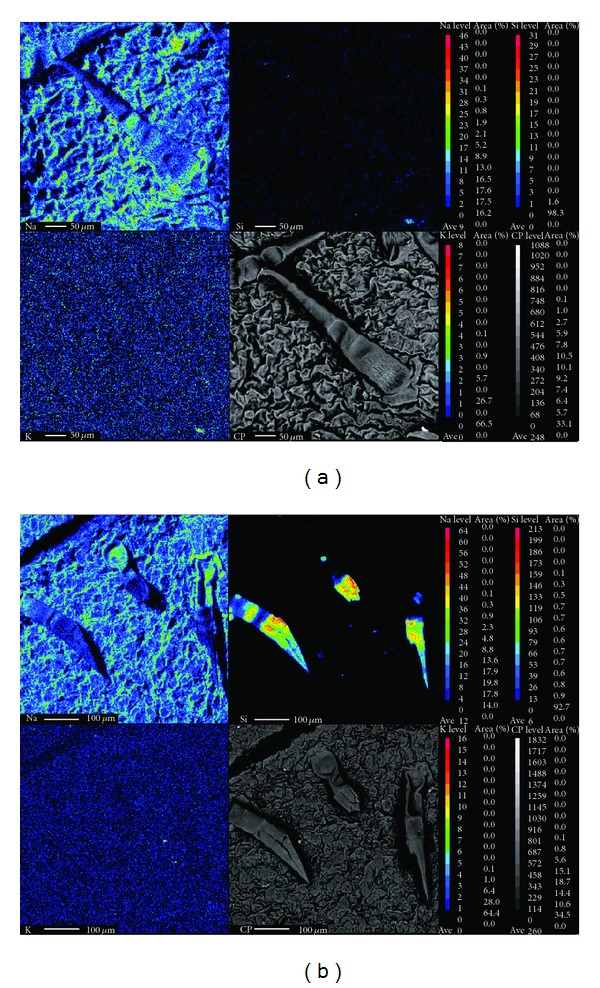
Wavelength dispersive X-ray analysis of Si-treated and nontreated leaves of *A. multiflora*. (a) MS medium and (b) MS + Si.
